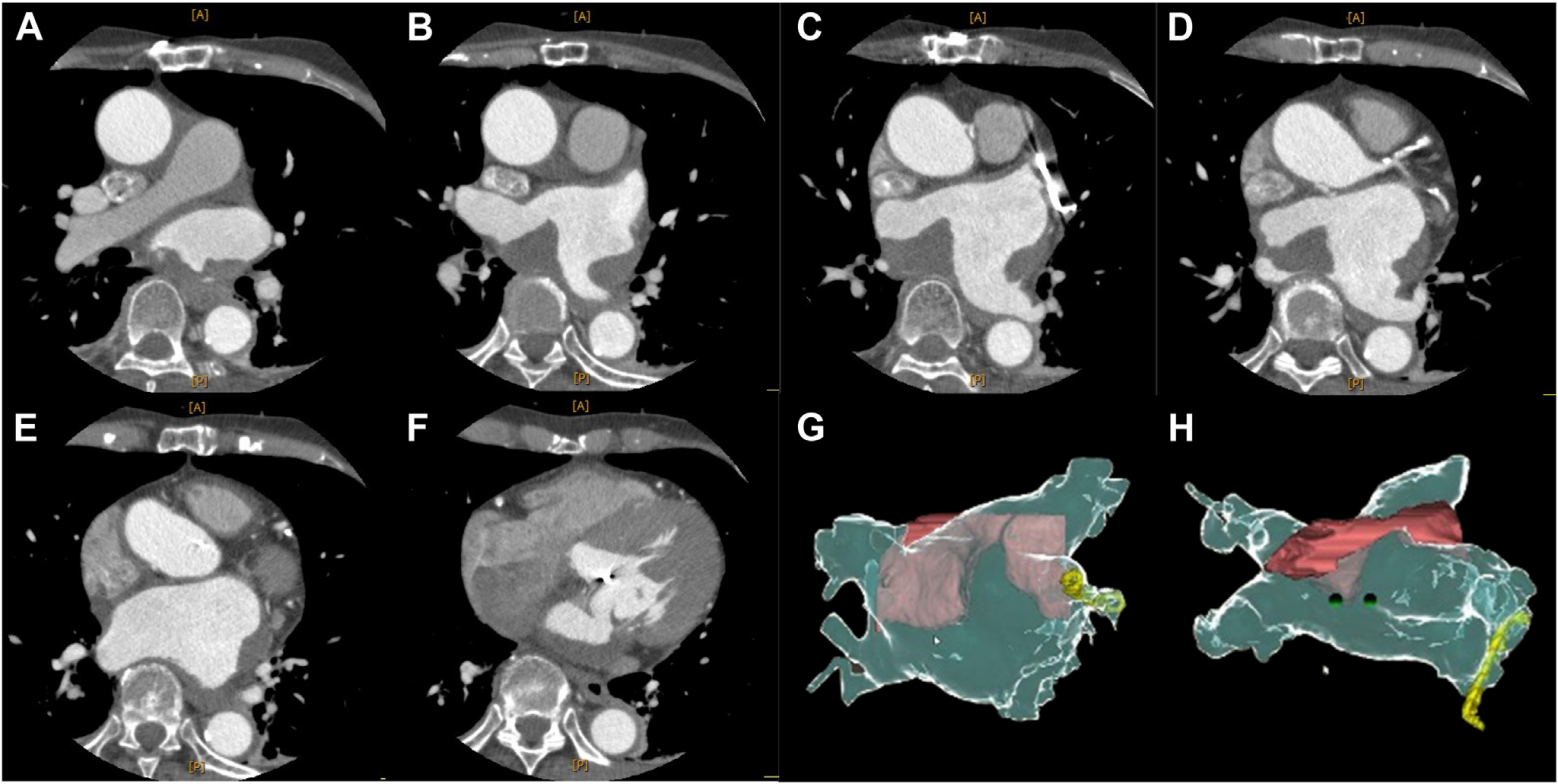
A–F: The CT images show that the thrombus is extensively attached from the posterior wall to the lateral wall of the LA. G, H: The 3D image of the thrombus: the red color represents the thrombus, extending from the posterior to the lateral wall. CT = computed tomography; LA = left atrium.
